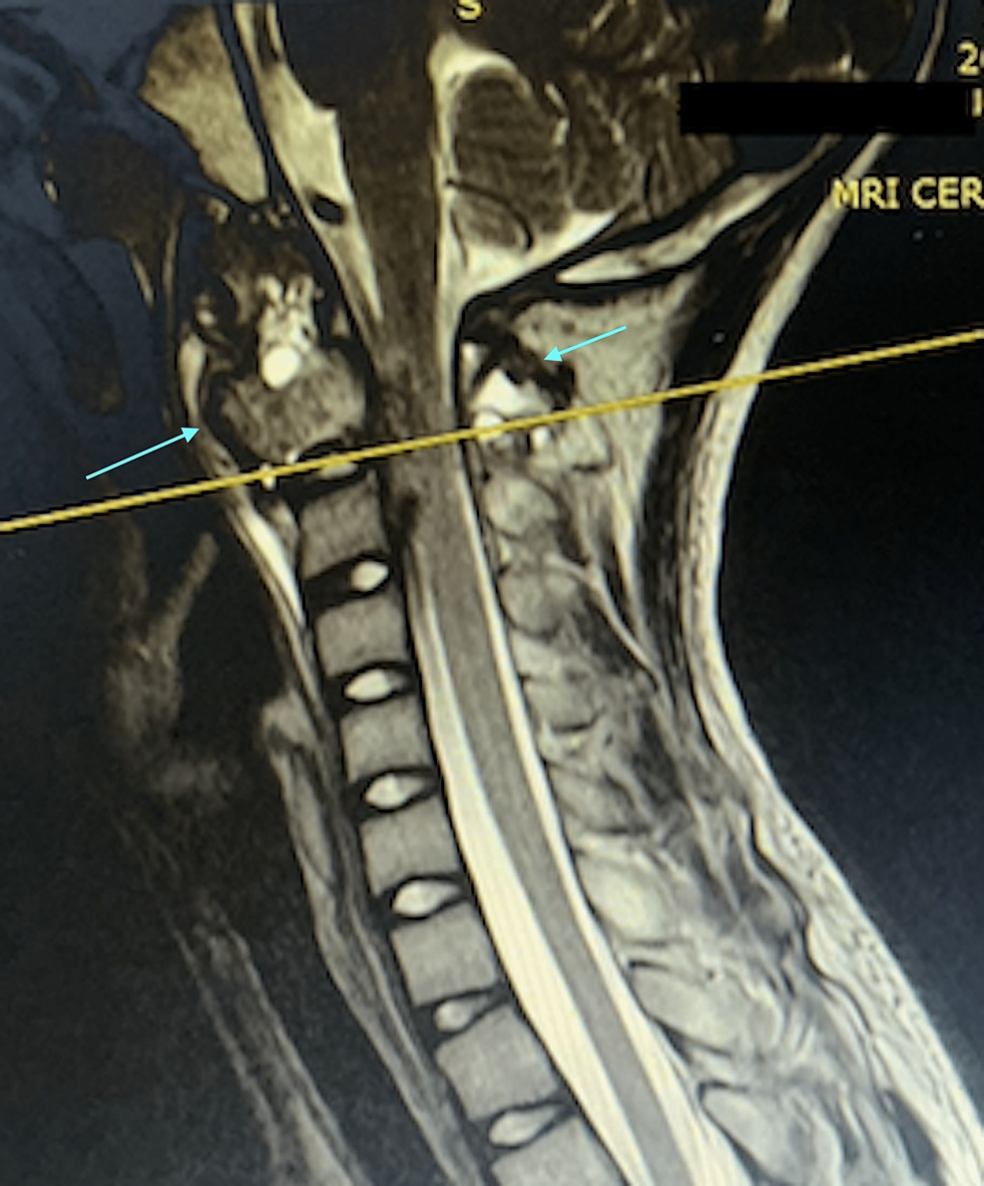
Sagittal MRI of the cervical spine This is the MRI from the time of clinical presentation. The light blue arrows show the lesion contained within the C2 vertebra and involving anterior and posterior columns.
